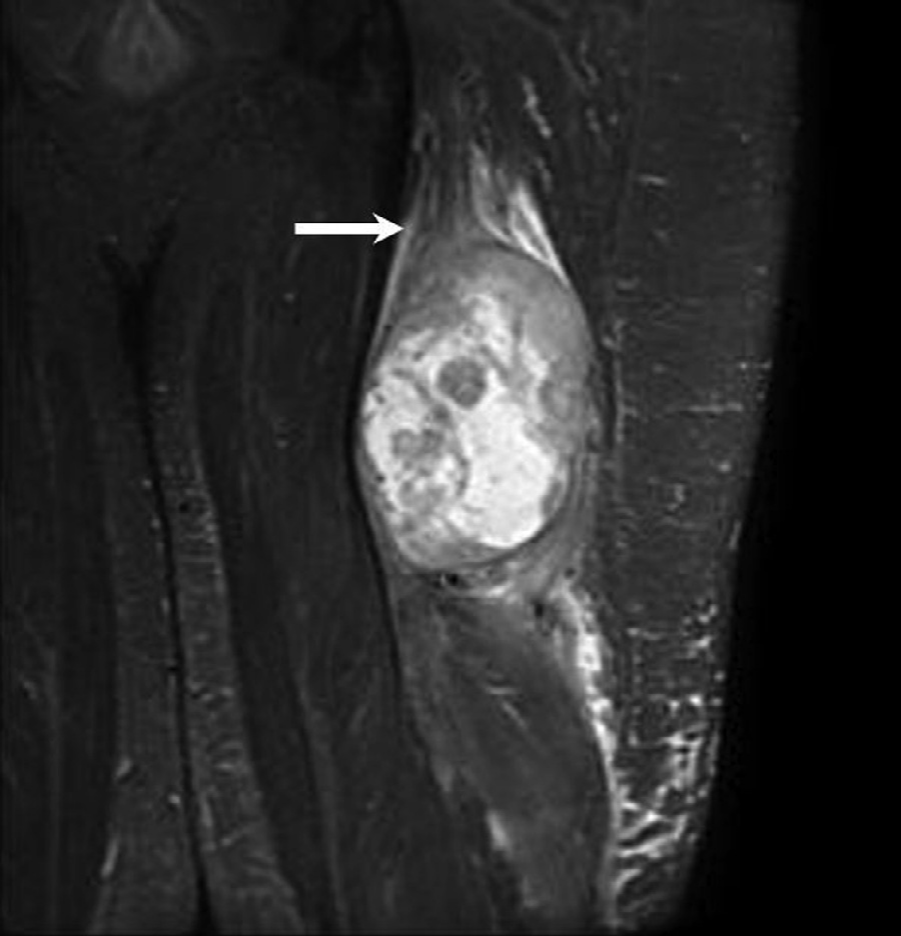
Coronal STIR MRI image shows a heterogeneous ovoid mass in the left thigh with adjacent edema. Proximally, it is continuous with the sciatic nerve (arrow).
